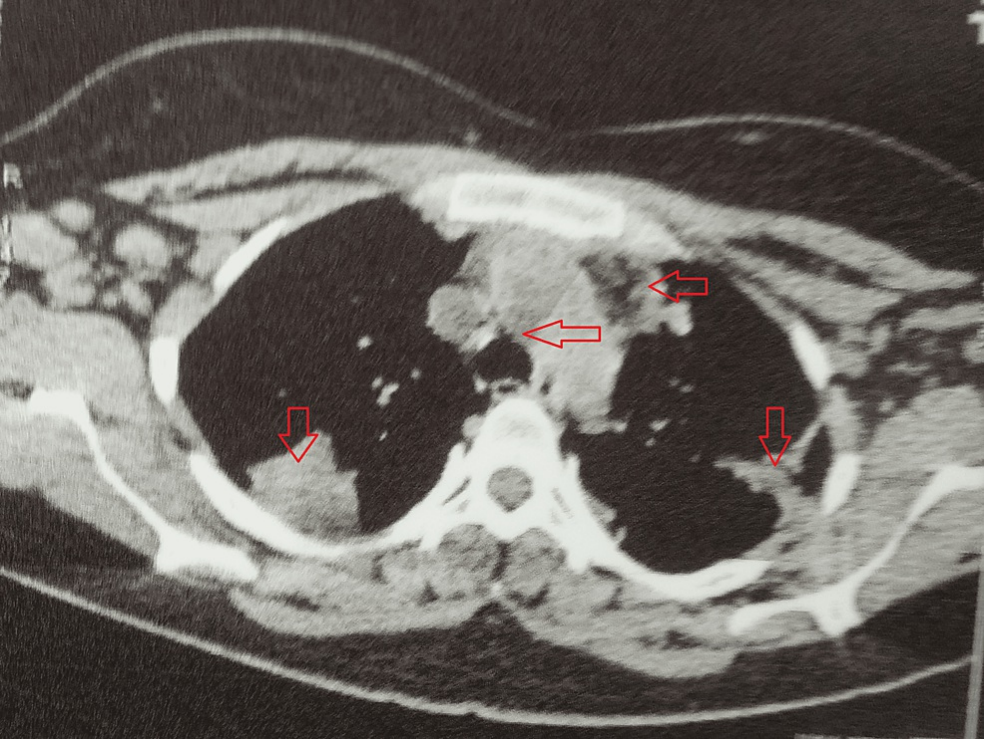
HRCT of the chest showing mediastinal lymphadenopathy and lung involvement HRCT: high-resolution computed tomography
